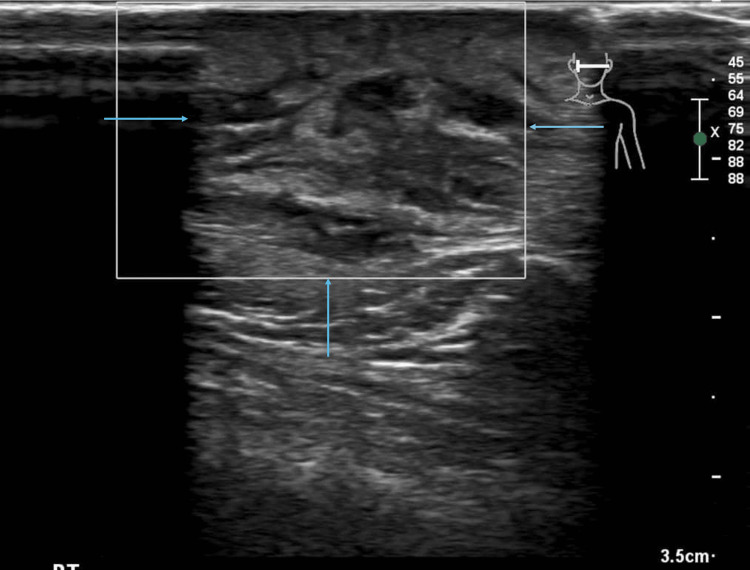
Follow-up ultrasonography of the lesion with color Doppler (June 2025) showing no significant intralesional vascularity (arrows), in contrast to the findings on the previous ultrasonography.
